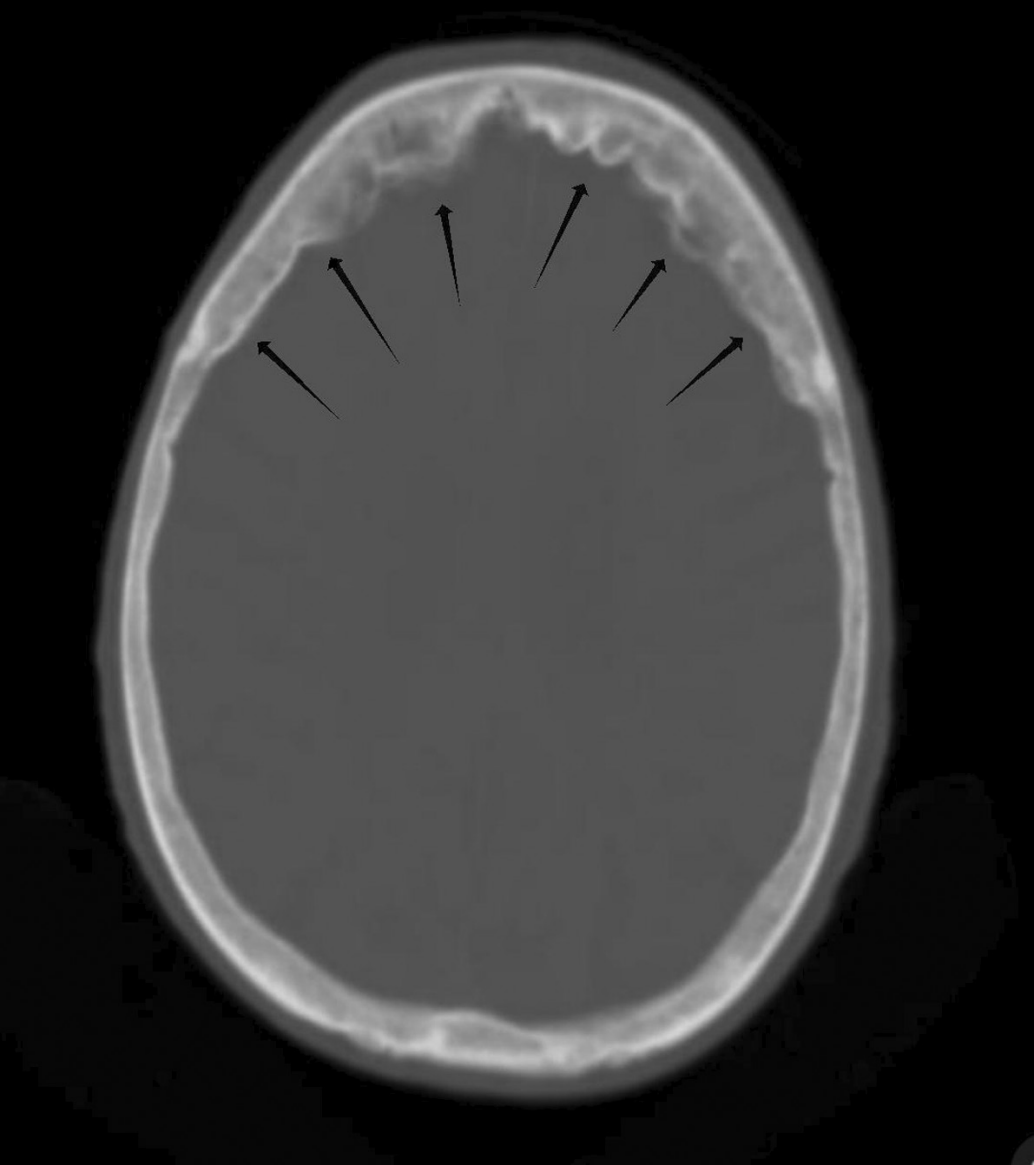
Non‐contrast axial computed tomography (CT) brain showing hyperostosis of inner table of frontal bone.
